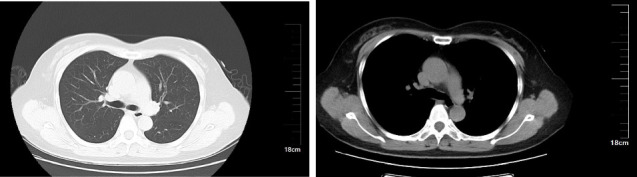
No significant abnormalities observed in chest CT for Case 2.
